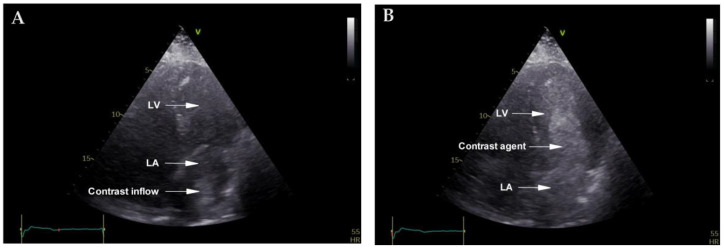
(A) Contrast transthoracic echocardiography, with an apical 4-chamber view showing contrast agent entering the left atrium via PLSVC drainage into the left inferior pulmonary vein. (B) Contrast transthoracic echocardiography, with an apical 4-chamber view showing early opacification of left atrium and left ventricle. Abbreviations: LA, left atrium; LV, left ventricle. N.B. The contrast agent was injected into the left arm.
